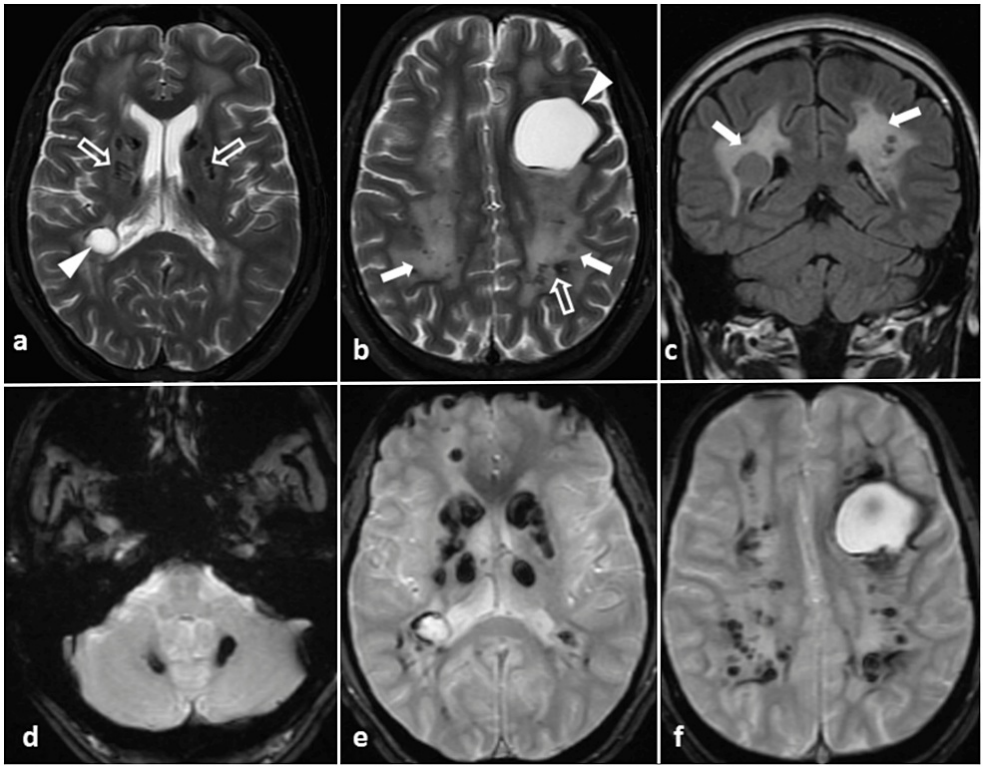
MRI of the brain done at the presentation: axial T2-weighted images (a and b) and coronal T2-FLAIR image (c) show confluent periventricular and deep white matter hyperintensity in both cerebral hemispheres suggestive of leukoencephalopathy (solid arrows) with cystic lesions (arrowheads) and T2 dark foci (hollow arrows). Gradient recalled echo images (d, e, and f) show near symmetric foci of blooming in bilateral dentate nuclei (d), bilateral basal ganglia (e), the white matter of both cerebral hemispheres and along the cyst walls (f). FLAIR: Fluid-attenuated inversion recovery.
